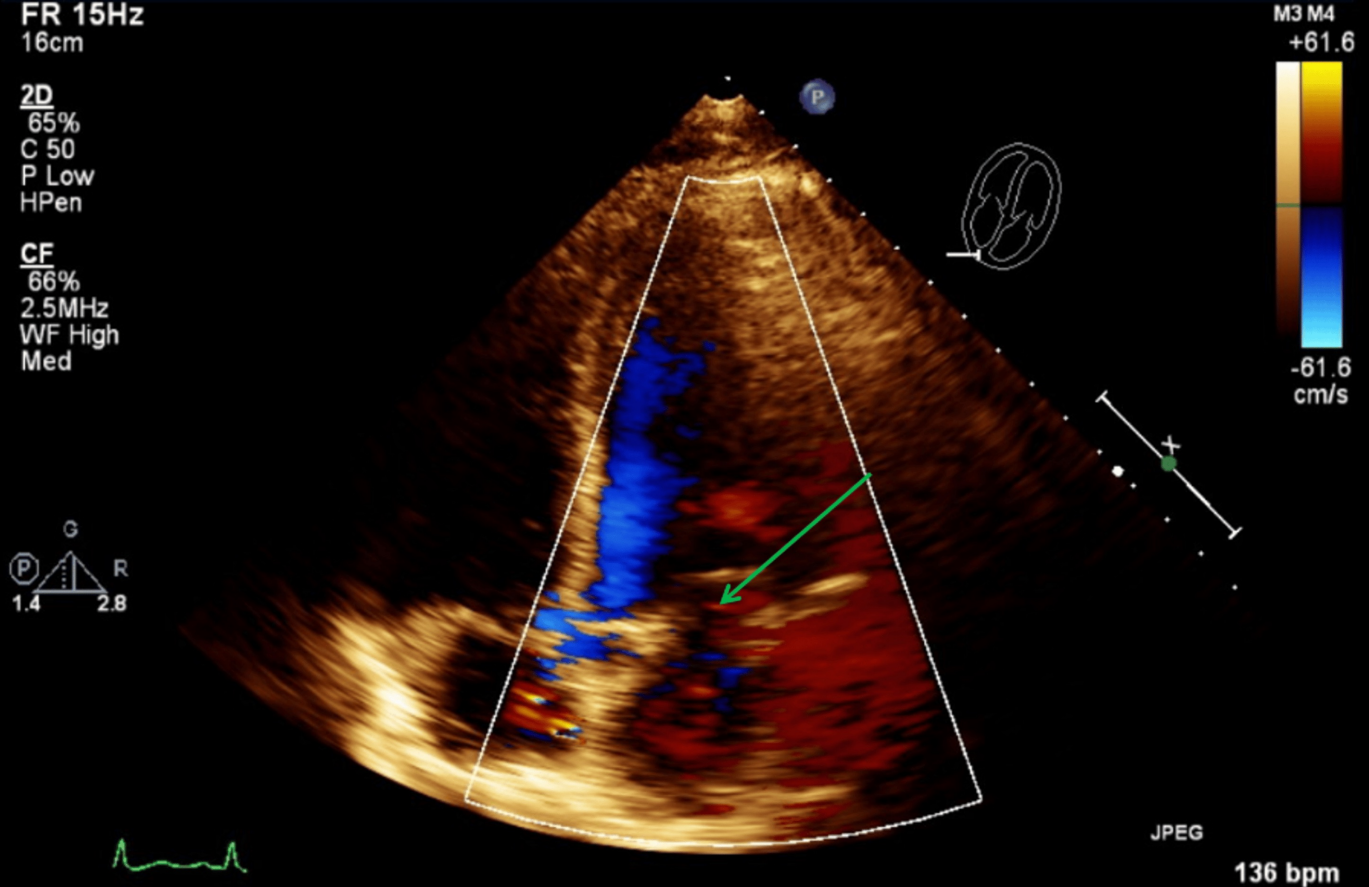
TTE apical three-chamber view with color Doppler during systole. The green arrow shows no findings of mitral regurgitation with color Doppler. TTE: transthoracic echocardiography
